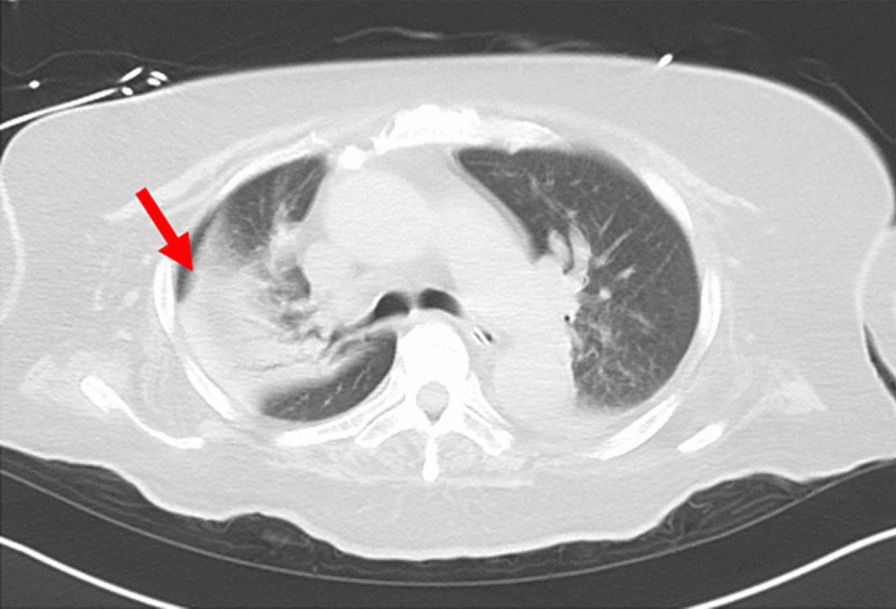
Computed tomography images of the chest. Red arrow indicates presence of atelectasis
